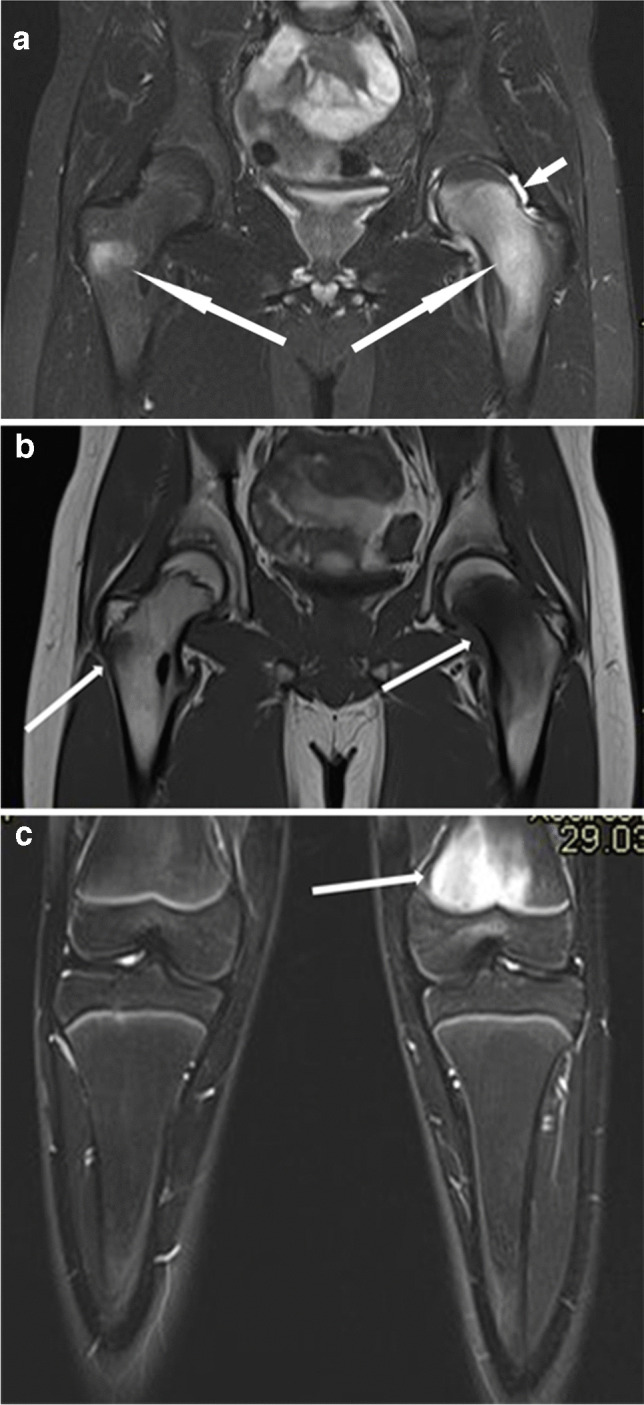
Magnetic resonance images in a 15-year-old boy with chronic recurrent multifocal osteomyelitis. a, b Coronal T2 turbo inversion recovery magnitude (TIRM) (a) and T1 (b) images of the hips show bone edema in the metaphysis and epiphysis of the left femur and at the base of the right greater trochanter (*long arrows*). There is a small reactive effusion in the left hip joint (*short arrow* in a). c Coronal T2 TIRM image shows bone edema in the distal metaphysis of the left femur (*arrow*)
